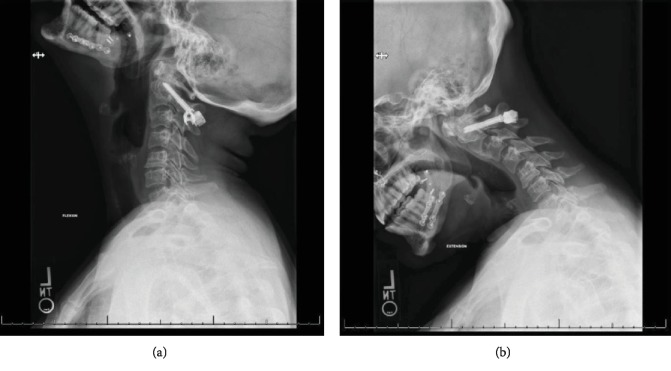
Radiographs of the cervical spine in extension (a) and flexion (b) display complete detachment of the tulip from the screw.
